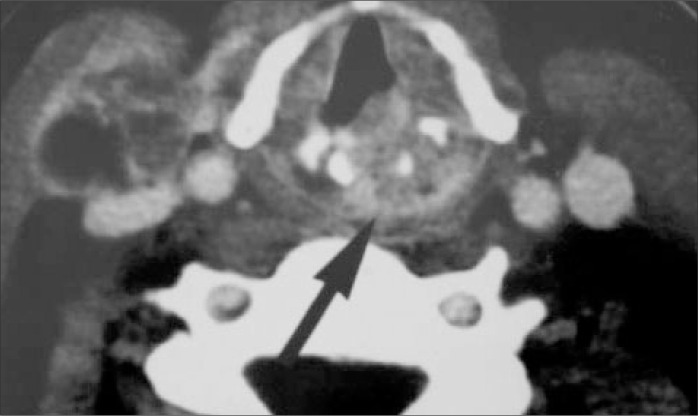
Axial computed tomography scan with lesion infiltrating posterior half of vocal fold, arytenoid cartilage and surrounding tissues (black arrow). Note the lymph node metastasis at level IV (right side).
